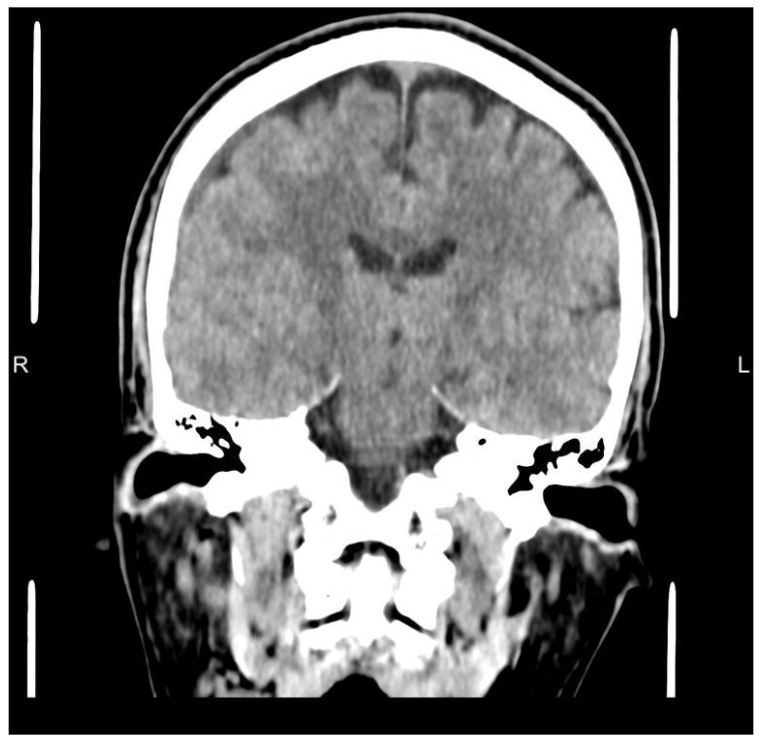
Follow-up non-contrast CT scan at eight months demonstrating near-complete resolution of the chronic subdural hematoma with normalization of midline structures.
